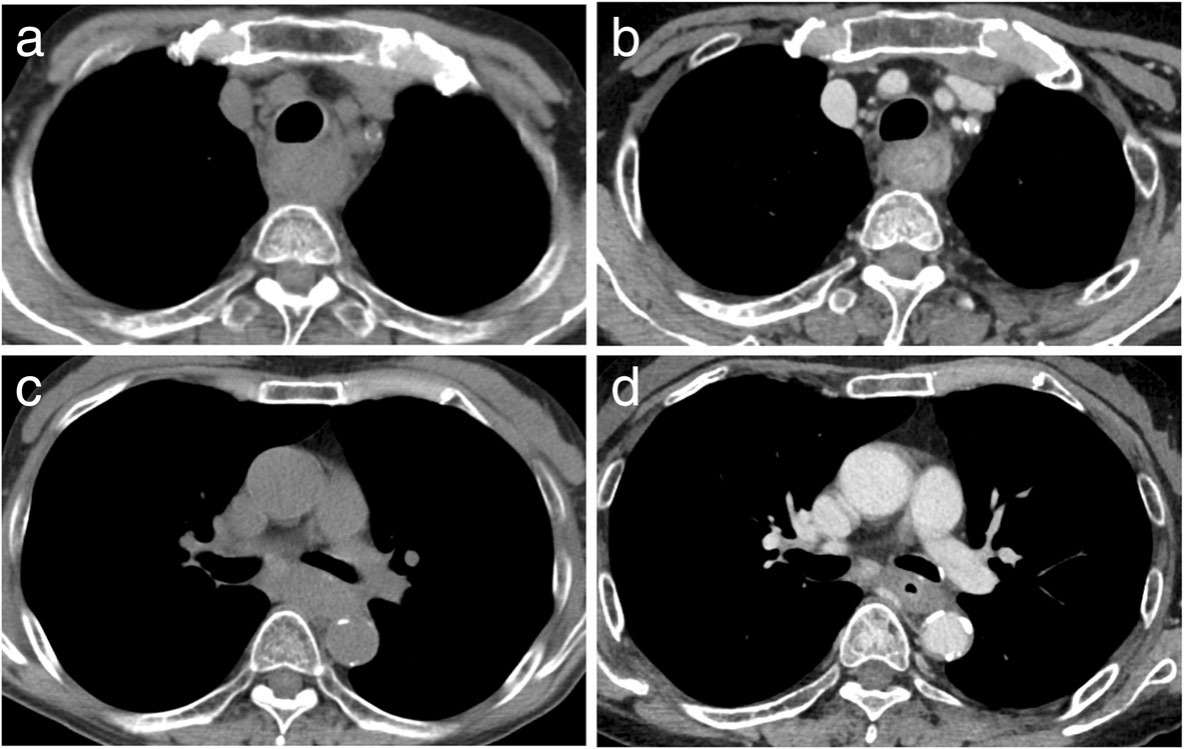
Computed tomography (CT) after AEN showed circumferential wall thickening of the upper to the lower thoracic esophagus: upper esophagus (a) and middle esophagus (b). Two years after AEN, a CT scan showed similar findings: upper thoracic esophagus (c) and middle thoracic esophagus (d)
